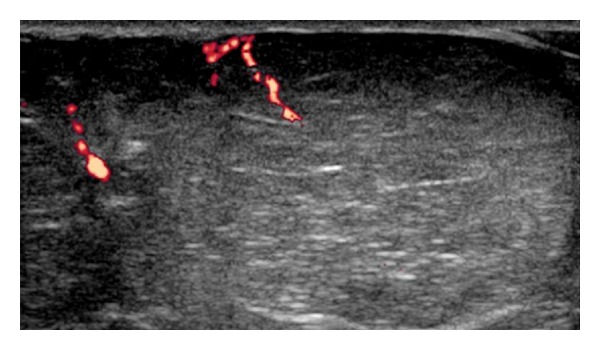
Ultrasound image of a superficial encapsulated lipoma with vascularity seen along the internal septae. The local reporting radiologist referred this lesion due to the internal vascularity. MDT determined the lesion benign on the basis of imaging and clinical information.
